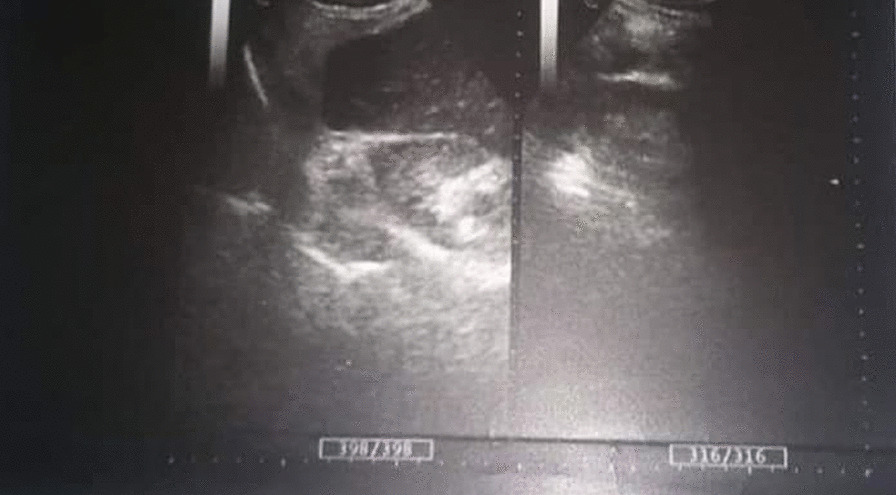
Abdominal Ultrasound demonstrating a normal liver and a resected gallbladder
